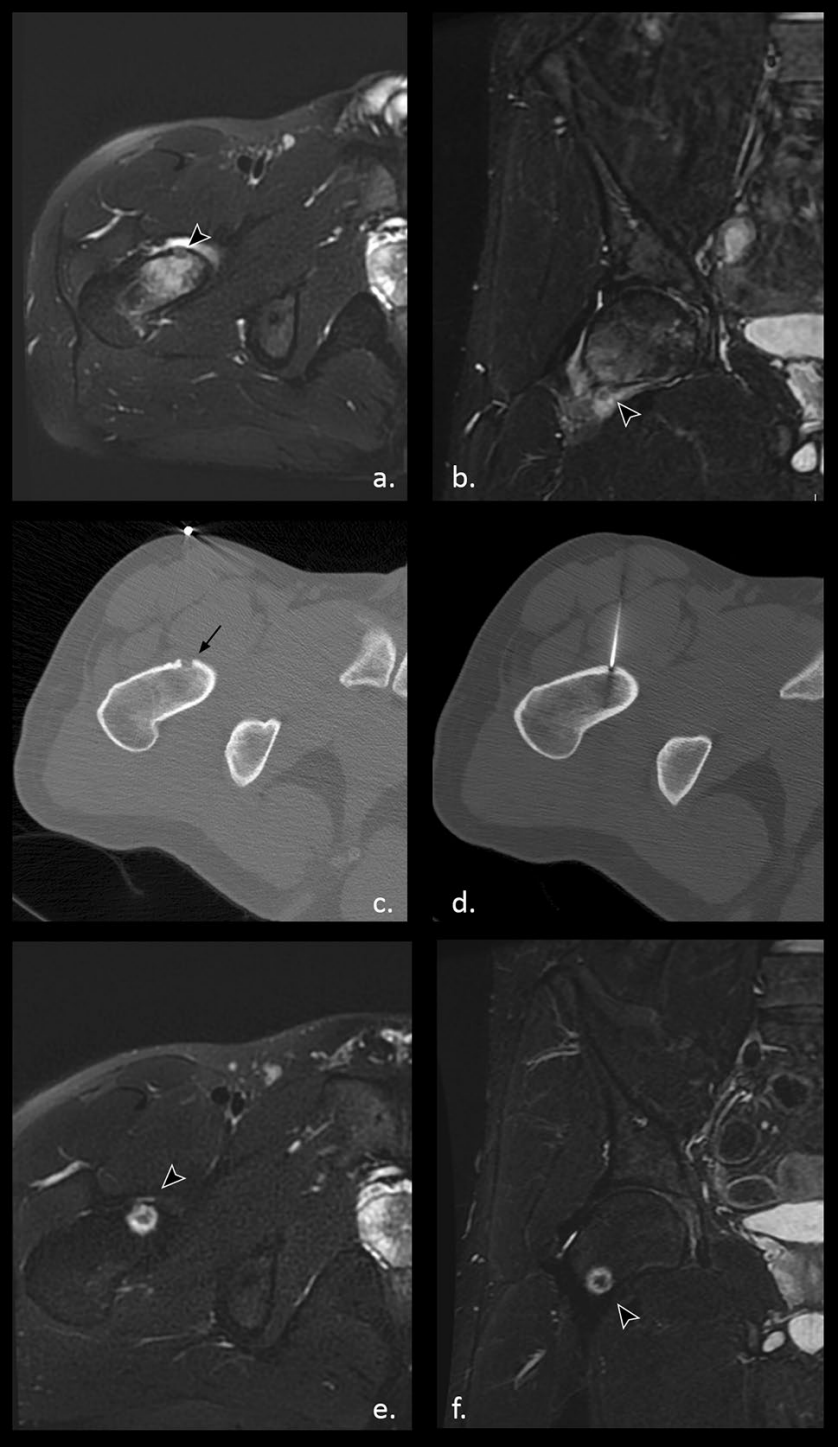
Osteoid osteoma of the right femoral neck. Axial (a) and coronal (b) MRI sequences weighted in STIR reveal the central nidus of the lesion (arrowhead) surrounded by extensive spongy bone edema and moderate joint effusion. c Axial CT scan shows the intracortical radiolucent nidus (black arrow) and the above cutaneous metal trace; d needle electrode positioning during radiofrequency thermal ablation procedure. Axial (e) and coronal (f) follow-up MRI sequences weighted in STIR after RFA demonstrate a sharp rim of post-procedural edema around the nidus
